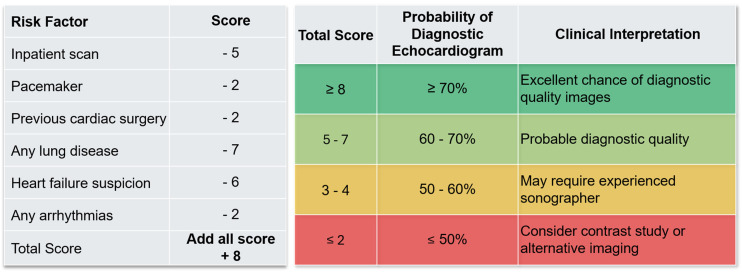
Clinical scoring tool for predicting the diagnostic quality of echocardiographic images based on patient-related risk factors. Panel (a) assigns negative scores to specific clinical factors influencing echocardiogram quality, generating a cumulative score (with baseline adjustment of +8). Panel (b) stratifies total scores into probability categories for diagnostic image acquisition and provides corresponding clinical interpretations, guiding decision-making for imaging modality selection.
